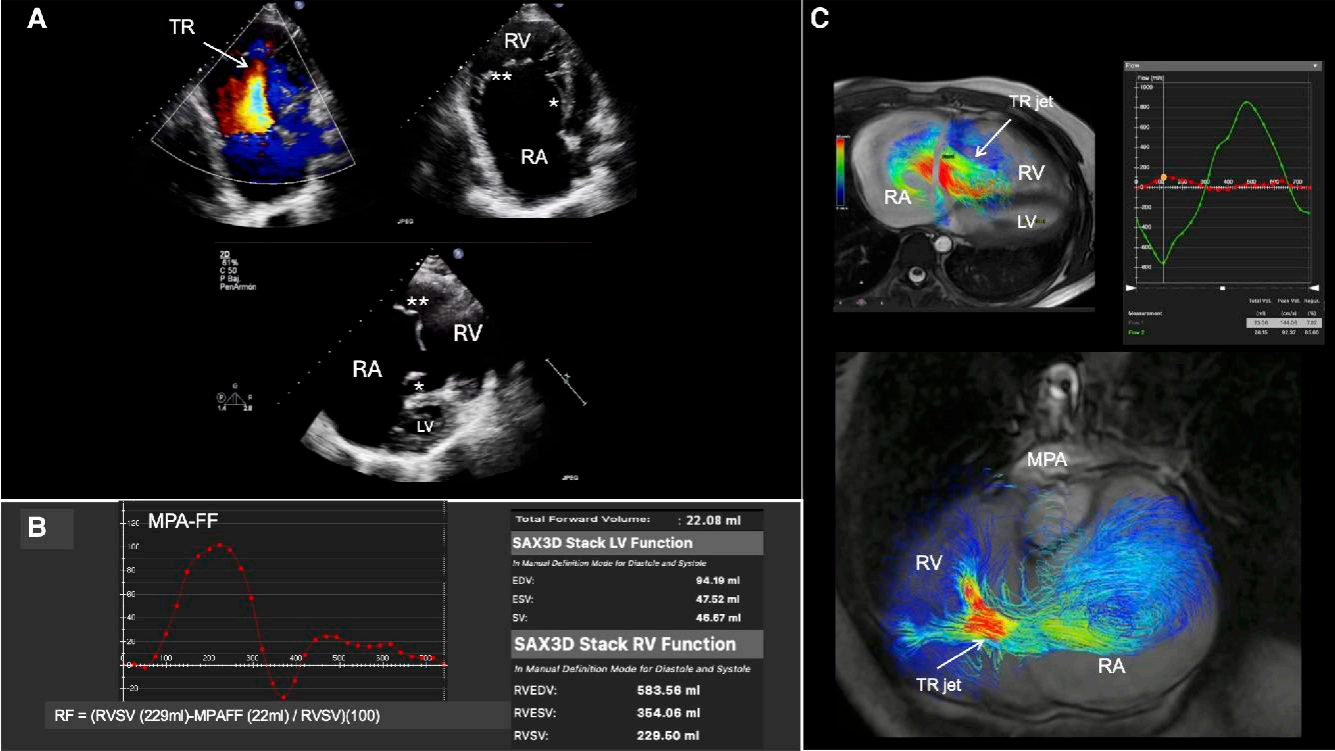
Panel *A*: 2D echocardiogram, RV focused images showing severe tricuspid regurgitation 8 (TR), short axis below.  Redundant anterior tricuspid leaflet. *Dysplastic septal leaflet. 9 Panel *B*: Main pulmonary artery 2D phase contrast forward flow and curve analysis. LV and 10 RV volumes are showed. 11 Panel *C*: 4D Flow images. 4 chamber view showing the torrential TR jet with a massive 12 vortex occupying the right atrium. Direct TR flow measurement at this level shows 85.6% TR 13 fraction. Torrential TR 3 chamber view.
